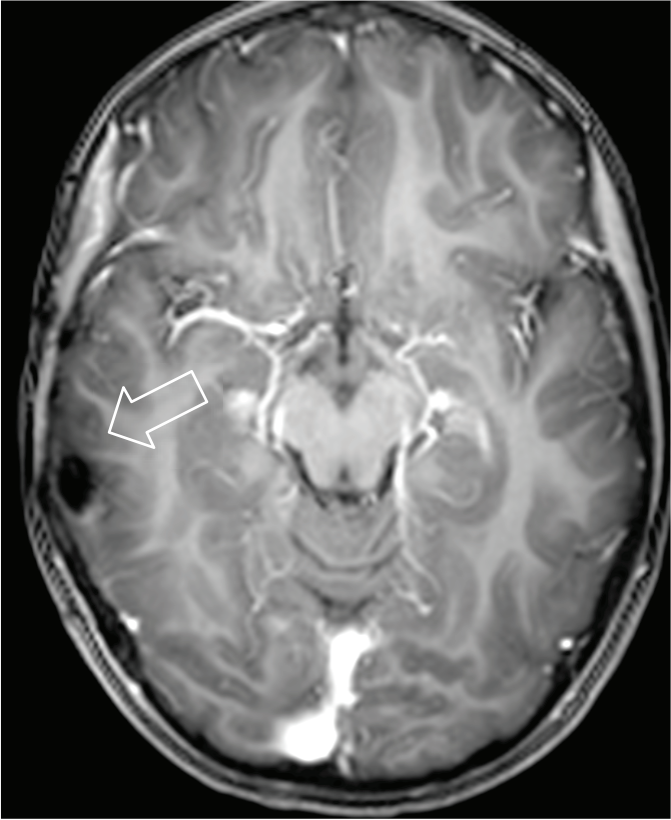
Axial MRI T1w + contrast. 2013 postoperative control. There are post-surgery changes and no evidence of residual lesion or abnormal enhancement.
